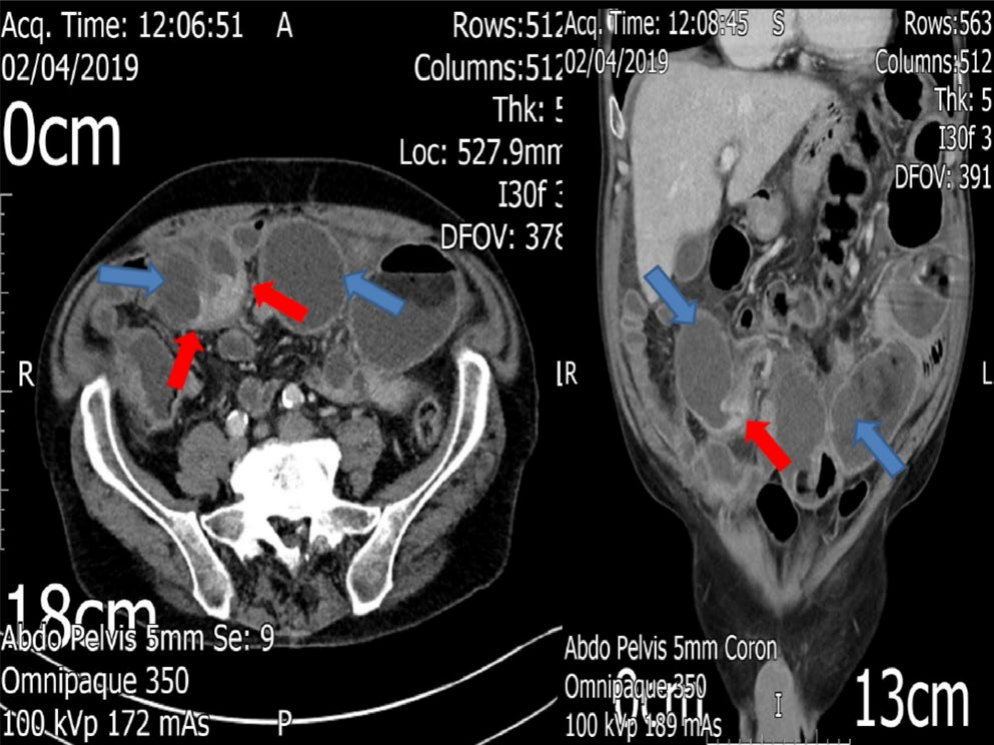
Axial and coronal views of the CT scan prior to the second operation; note the presence of the stenosis of Garré in the previously herniated small bowel segment, with fibrotic appearances and luminal narrowing (red arrows), causing gross prestenotic small bowel dilatation (blue arrows)
